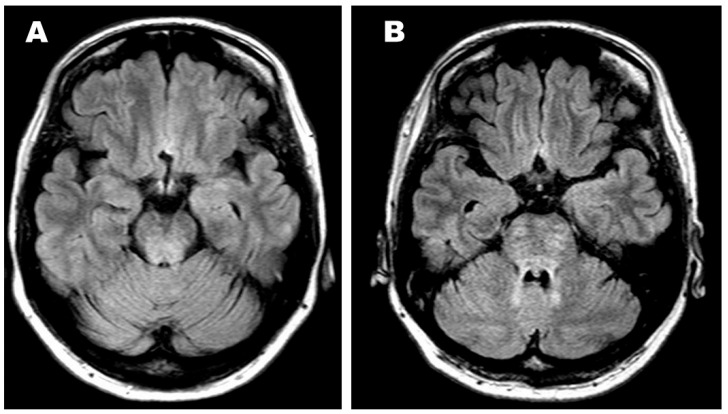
MRI shows a pons lesion. (A) At the diagnosis, when histiocytosis was not suspected. (B) After 7 months of Dab/Tra therapy for mixed histiocytosis (LCH/ECD).
